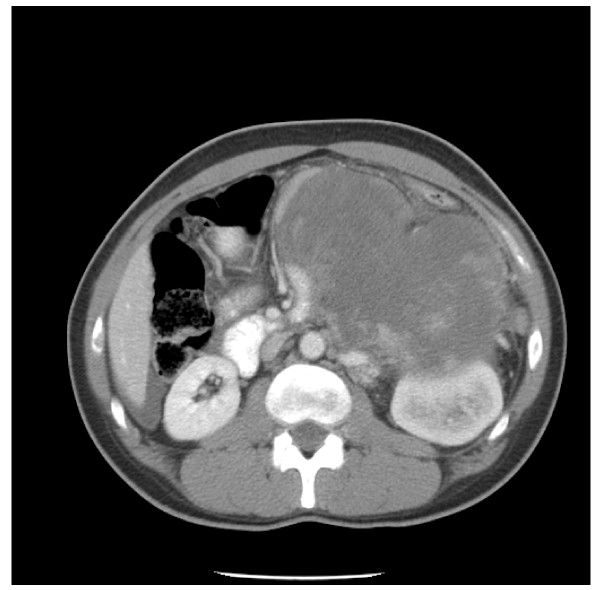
CT scan of adrenocortical carcinosarcoma. Necrotic left adrenal mass in close proximity to the body/tail of pancreas and third portion of the duodenum with displacement of the kidney
